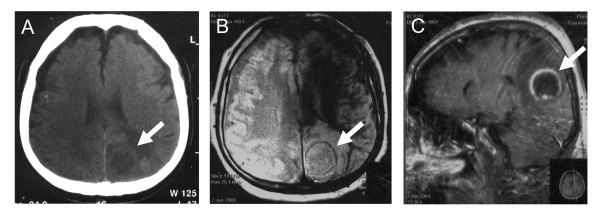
A 53-year-old man with reported seizures was evaluated. (A) Head CT without contrast medium reveals a round left occipital mass lesion (arrow) with hyperdense margins and a hypodense center. (B) A T-2-weighted head MRI image without contrast shows a mass (arrow) with high central signal intensity, a ring of heterogeneous peripheral signal intensity similar to that of the brain parenchyma, and a surrounding area of bright signal in the white-matter tracts. (C) On the contrast-enhanced T-1-weighted head MRI image, the mass has low signal intensity centrally that suggests the presence of fluid, and is surrounded by an enhancement ring, beyond which extends an area of low signal that indicates edema.
